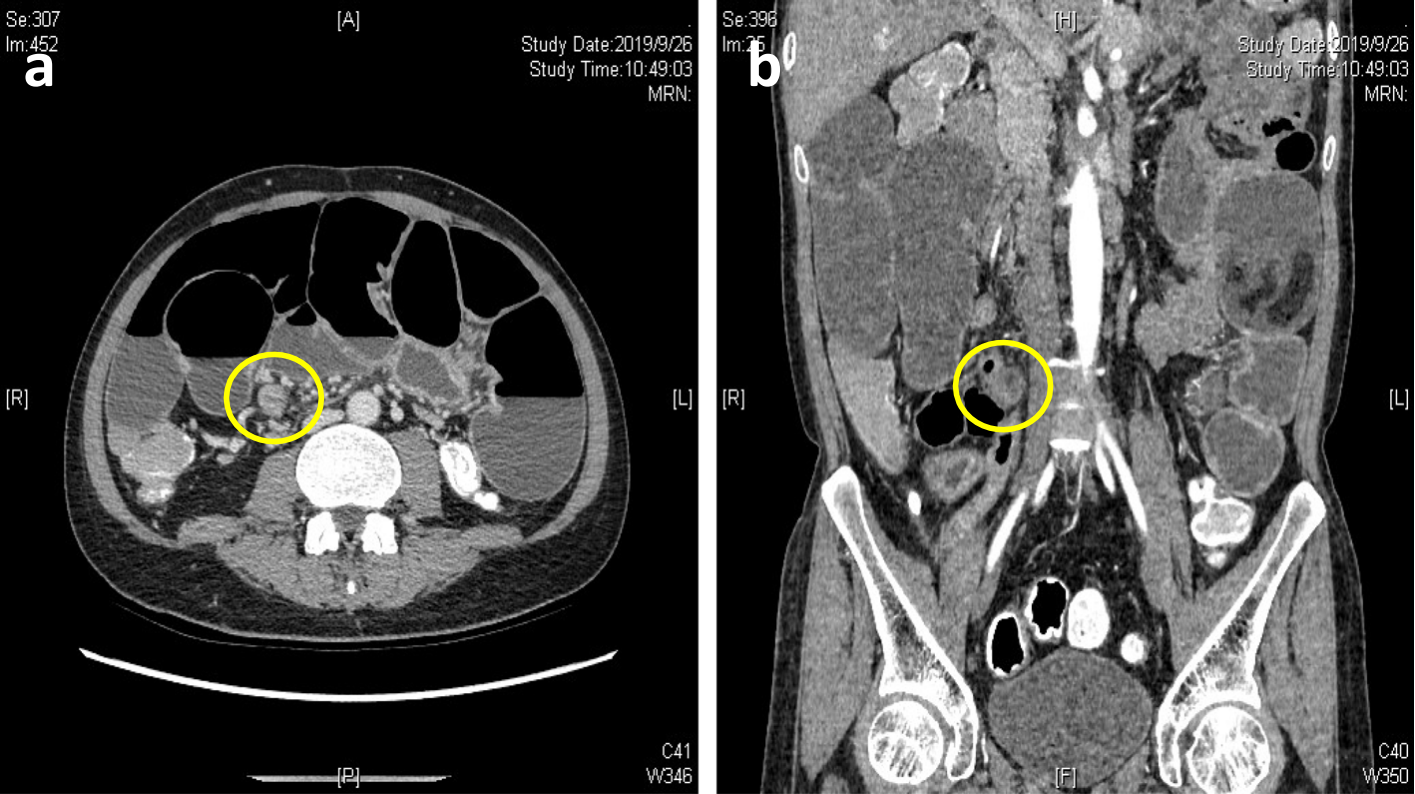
CT scan demonstrating yellow circle indicates the abrupt narrowing zone of the jejunum (a; cross-sectional image, b; coronal image)
